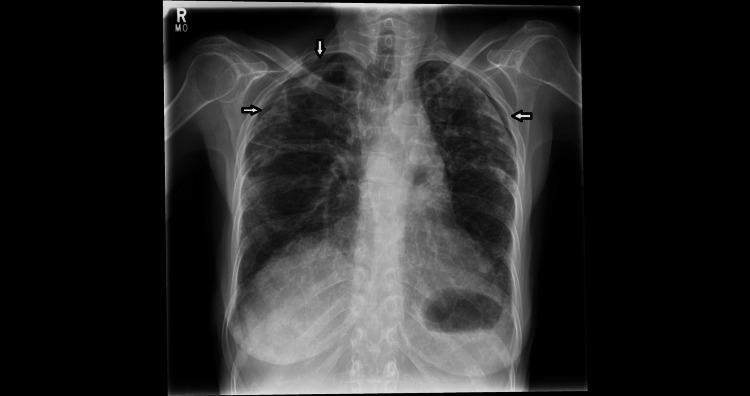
There is bi-apical pleural thickening, pleuro-parenchymal thickening, upper lobe volume loss and superior retraction of the hila. Note the small bilateral apical pneumothoraces in the CXR on admission (white arrows). CXR: chest X-ray
